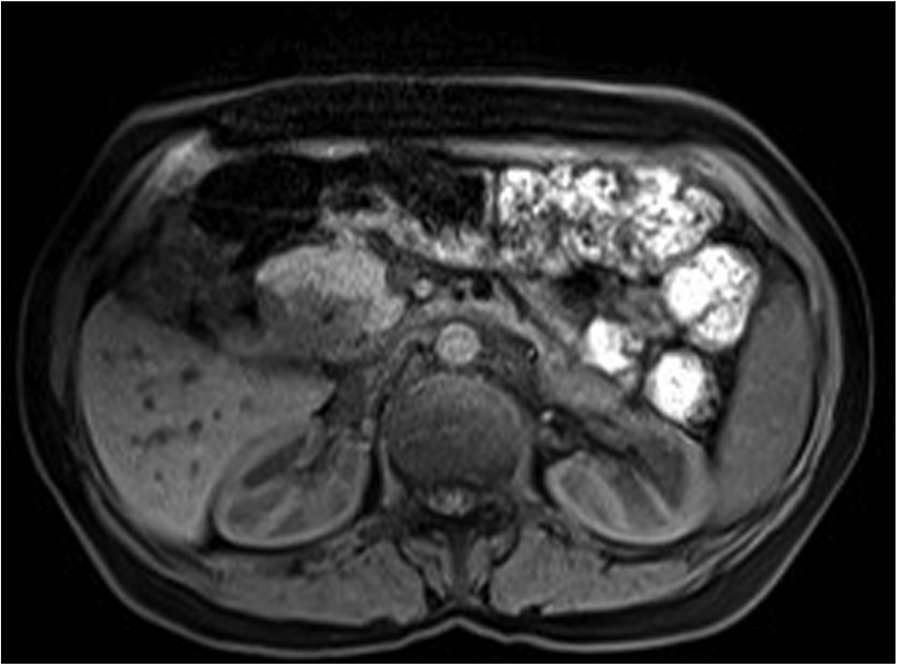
Contrast-enhanced T1-weighted MRI shows a hypointense lesion in the periampullary region near normal hyperintense pancreatic tissue
